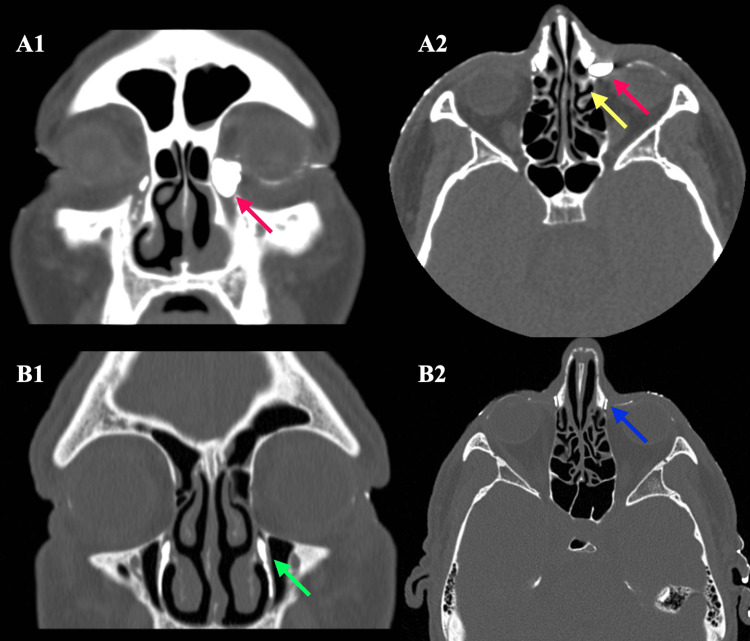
CT scan with iodine contrast enhancement of the lacrimal pathway. (A) Lower nasolacrimal duct obstruction (A1: coronal view; A2: axial view) resulting in a mucocele of the left lacrimal sac. (B) Contrast medium correctly draining onto the inferior lacrimal pathways (Hasner’s valve). As no signs of lower nasolacrimal duct obstruction are visible, different causes of epiphora should be investigated (B1: coronal view; B2: axial view). Red arrow: mucocele of the lacrimal sac; yellow arrow: insertion of the uncinate process; green arrow: inferior lacrimal drainage pathway; blue arrow: common canaliculi.
